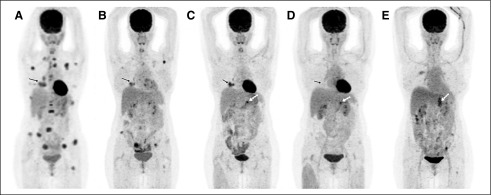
Maximum intensity projection images of (A) initial staging positron emission tomography (PET; August 2016) revealed an [^18^F]fluorodeoxyglucose (FDG)-avid soft tissue lesion in the right lower lobe (RLL; arrow) along with FDG-avid mediastinal/supraclavicular lymph nodes and multiple lytic bone lesions (appendicular and axial skeleton). (B) Follow-up PET (November 2016) depicted interval decrease in the size of the RLL lesion (arrow) and metabolic activity of the skeletal lesions. (C) Follow-up PET (March 2017) revealed an increase in metabolic activity of the RLL lesion (arrow) and appearance of a new FDG-avid left adrenal nodule (dashed white arrows). (D) Follow-up PET (September 2017) showed complete resolution of the RLL lesion and decrease in FDG avidity of the left adrenal nodule (dashed white arrow). (E) Follow-up PET (September 2018) revealed interval progression in the metabolic activity of the left adrenal nodule (dashed white arrow) and new-onset FDG-avid lesion in the left femur.
